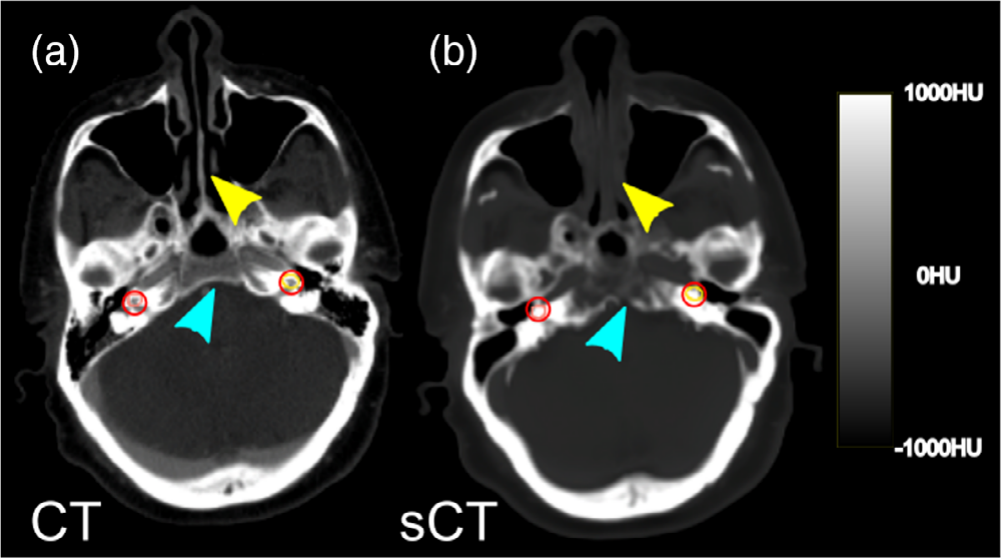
Example of tissue misclassification for the cochleae (red circle) in CT (a) and sCT (b). The cochleae are small volume structures that sit near bone, air and soft tissue interfaces and had HU differences > = 80 HU for 79% (left cochlea) and 85% (right cochlea) of the tested patients. An example of soft tissue defined in air is indicated with the yellow arrow and soft tissue as bone by the blue arrow.
